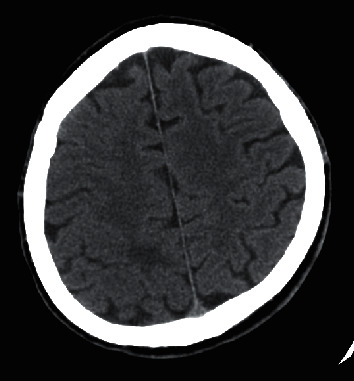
Axial CT image of the brain with a hypodense lesion in the right parietal lobe, surrounded with oedema.
